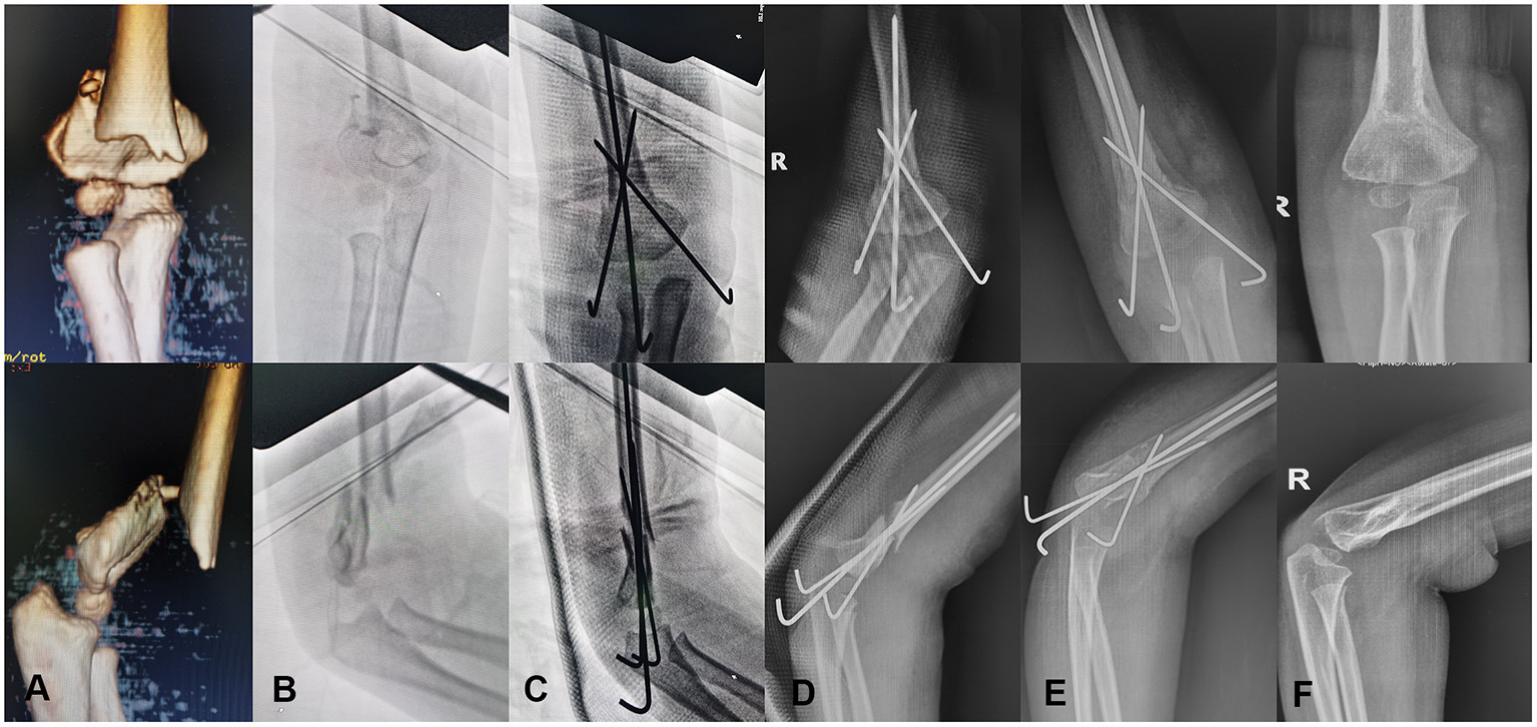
A 4-year-old boy with displaced MDJ fracture of his right elbow. (A) The coronal and sagittal 3D-CT images of the fracture; (B) the AP and lateral radiographs of the fracture before intraoperative reduction; (C) the AP and lateral radiographs after the utilization of the novel CRPP procedure intraoperatively; (D) the AP and lateral radiographs at 1 day after surgery; (E) the AP and lateral radiographs at the time of pin removal 4 weeks post-operatively; (F) the AP and lateral radiographs at 12 months post-operative follow-up.
